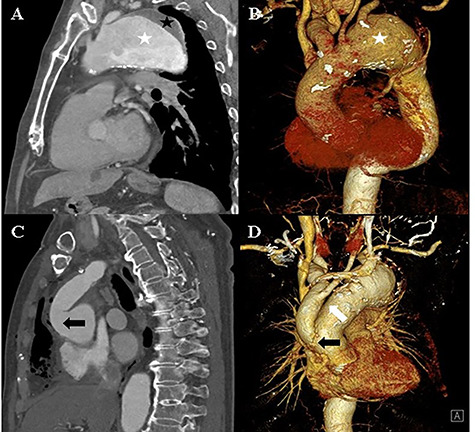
A and B: Computed tomography of the chest shows an aortic aneurysm (white asterisk) with a mural thrombus (black asterisk) at the distal arch. C and D: Post-debranching operative computed tomography of the chest shows the graft, which is directly sutured onto the ascending aorta (black arrow), and the graft used from the innominate artery to the left common carotid artery (white arrow).
